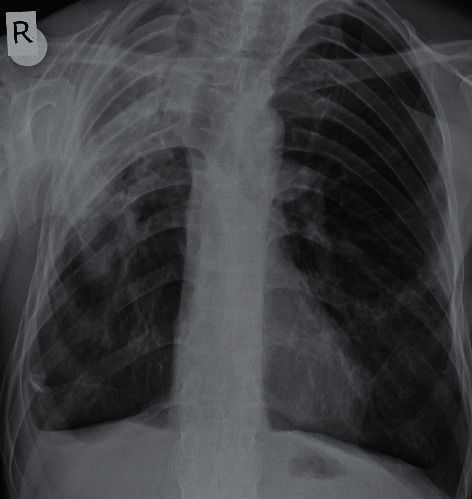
Chest X-ray shows diffuse opacities in the upper zone of the right lung. Also, it reveals deviation of the trachea to the right side and blunting of the right costophrenic sinus.
